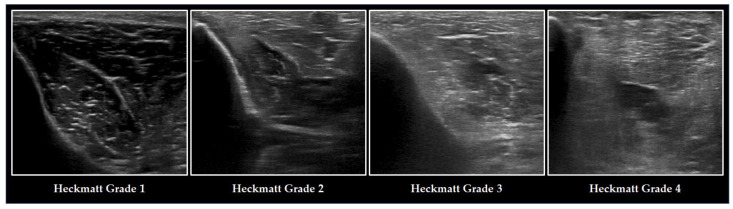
Ultrasonographic changes of the TA and the adjacent tibia in ICUAW graded with the Heckmatt Scale. The image on the left refers to a healthy individual, graded with lowest Heckmatt grade 1. Muscle tissue is classically dark with some bright echo signals from connective tissue. In critical illness (from the second left image) and especially in patients with ICUAW (from the second right image), muscle tissue becomes more echointense (Heckmatt grade 2) and the bone echo begins to become blurry (Heckmatt grade 3), until it has nearly vanished (Heckmatt grade 4).
